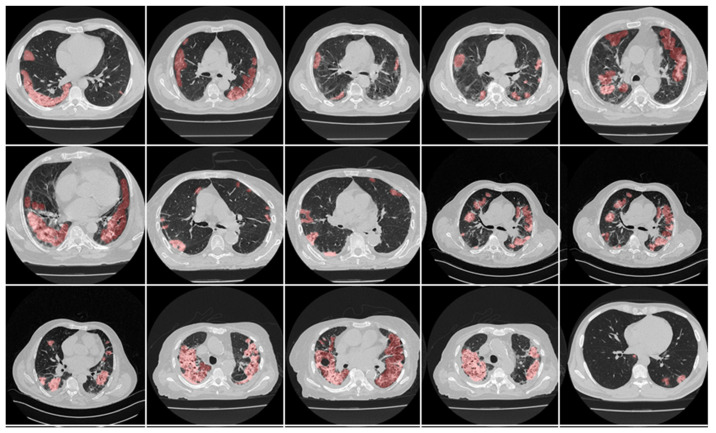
Manual delineation overlays (red) from tracer 2 on raw CT images.
